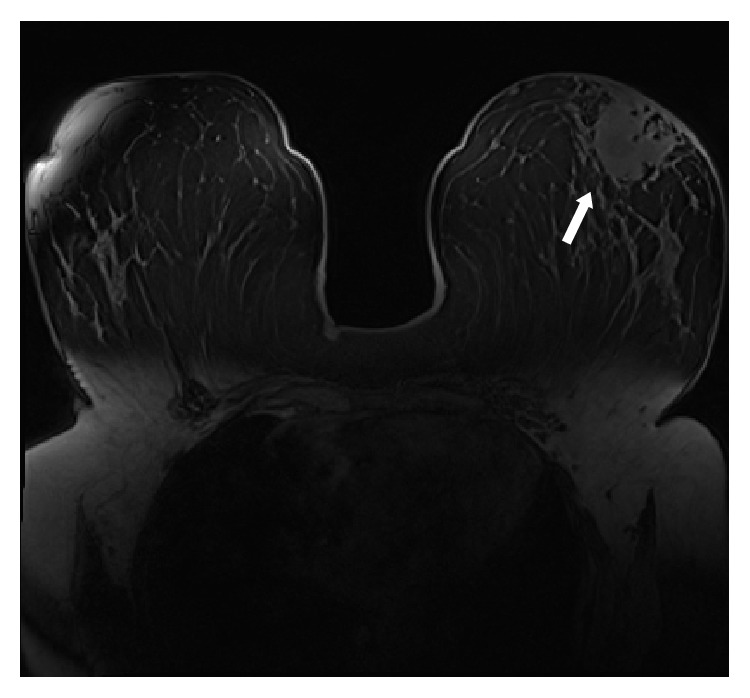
Granulomatous mastitis left breast. T1 axial postgadolinium MRI demonstrating retroareolar enhancing abnormality with periareolar skin thickening. It cannot be distinguished from a malignancy based on these imaging findings.
